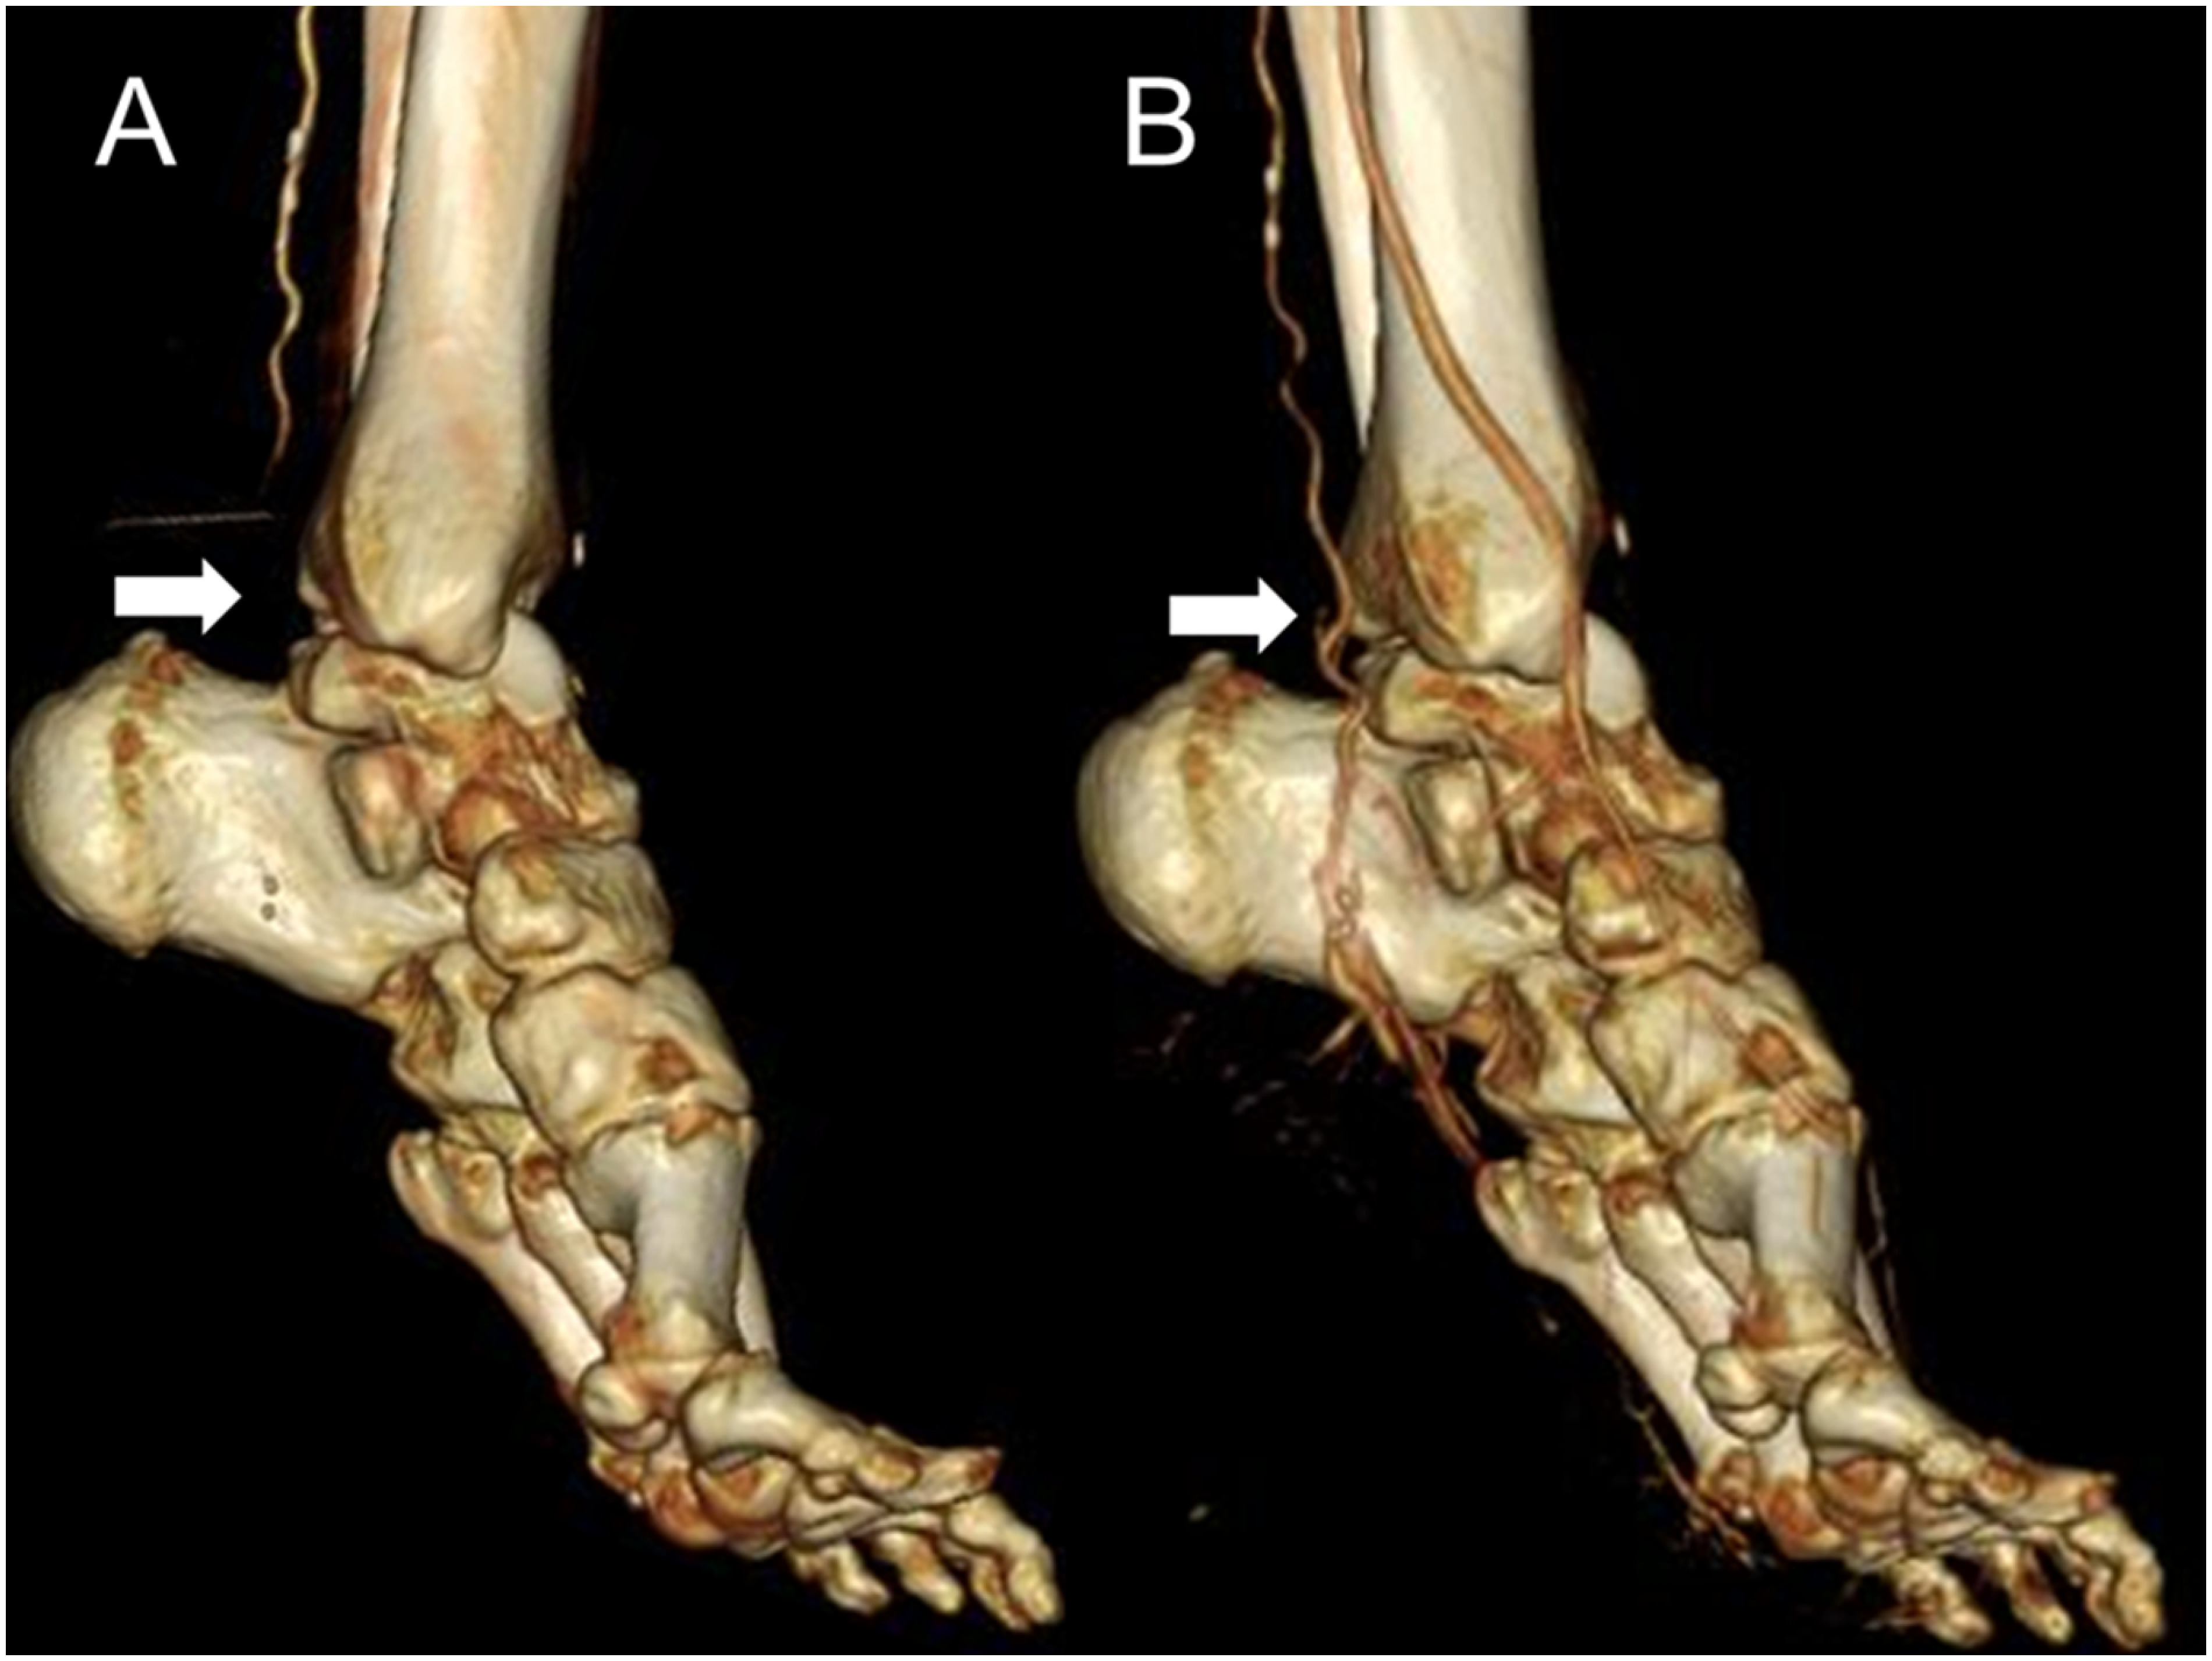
Fig. 2 (A) Preoperative computed tomography (CT) angiography showing a patent posterior tibial artery. The plantar artery and the dorsal artery at the level of the ankle are occluded. (B) Postoperative CT angiography showing that the common plantar artery is patent.
